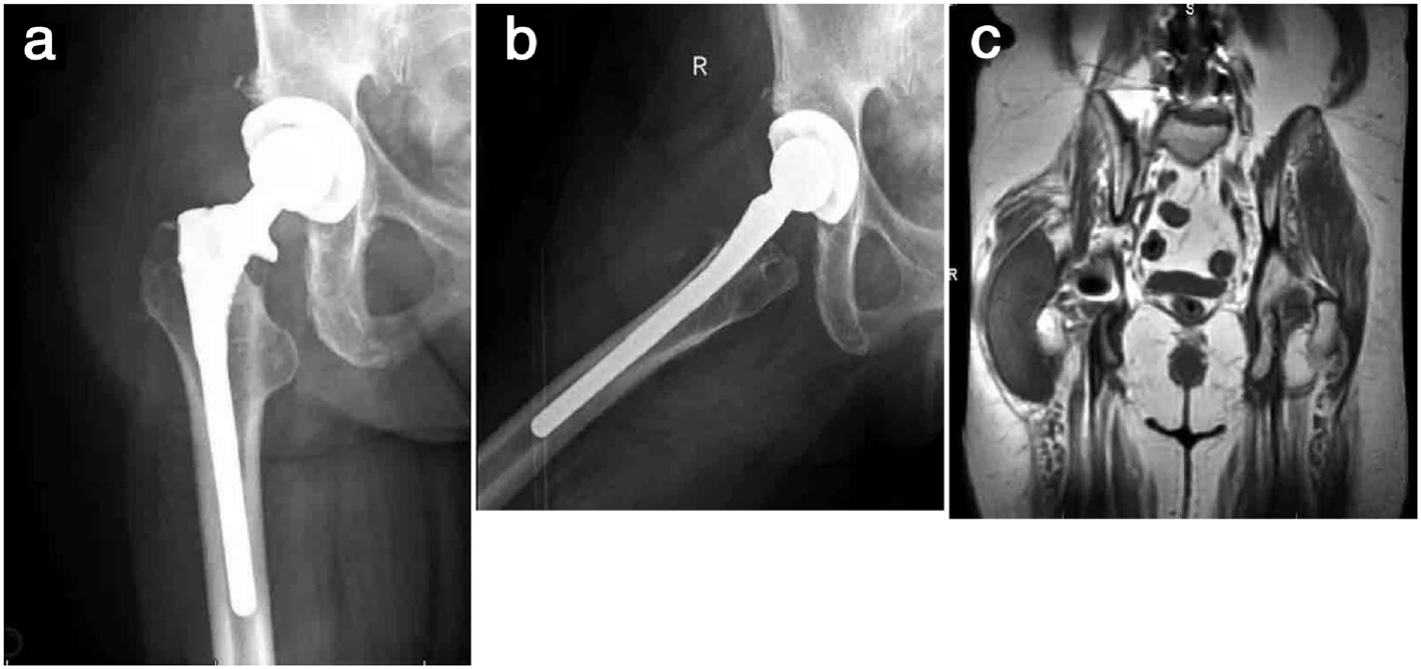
a, Anteroposterior and b, lateral radiographs demonstrating an uncemented total hip arthroplasty. c, Coronal T1-weighted MRI cut demonstrating the large well-circumscribed lesion overlying the right hip joint.
